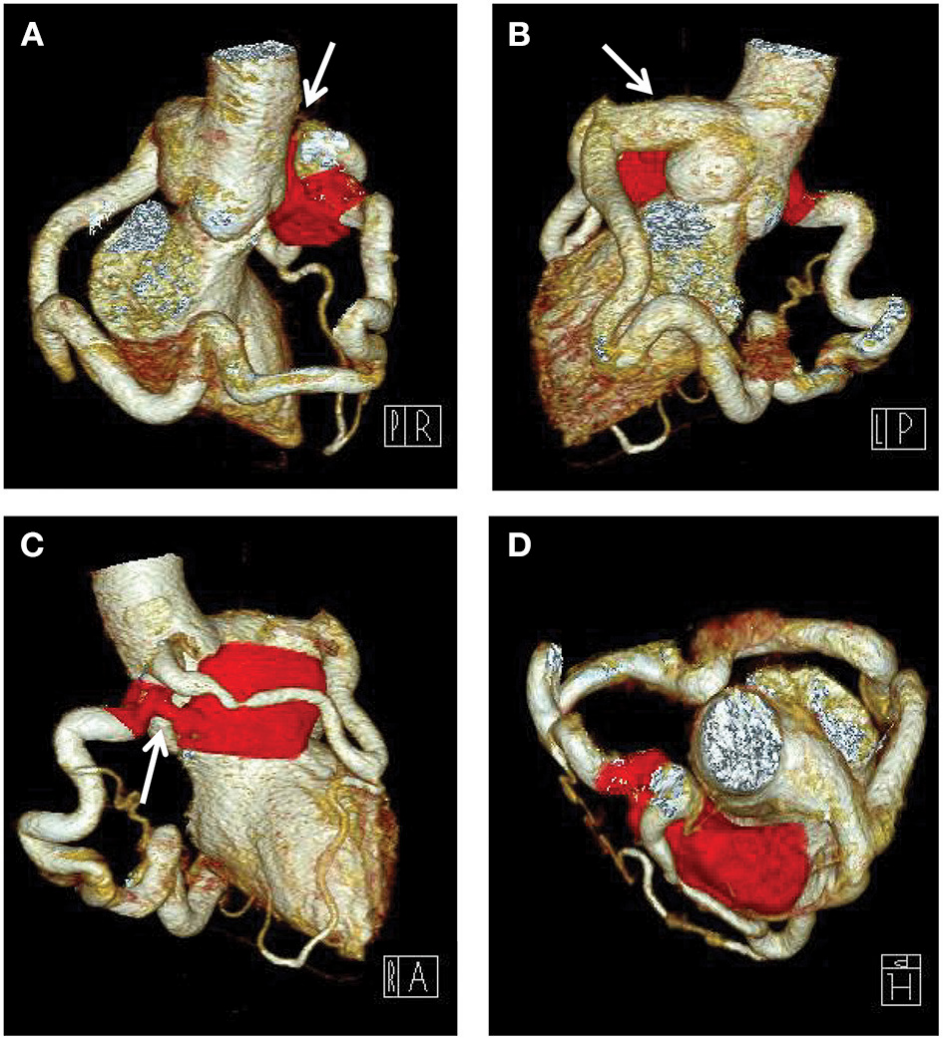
Computed tomography angiography preoperatively showing that there is an interruption between right sinus of Valsalva and the enlarged and tortuous right main coronary artery (A, arrow), a single left coronary ostium with a giant coronary trunk (B, arrow), a 7-mm fistula into the right ventricle (Red) (C, arrow), the left and right coronary artery were connected with each other and formed a closed loop at the base of the heart (D).
